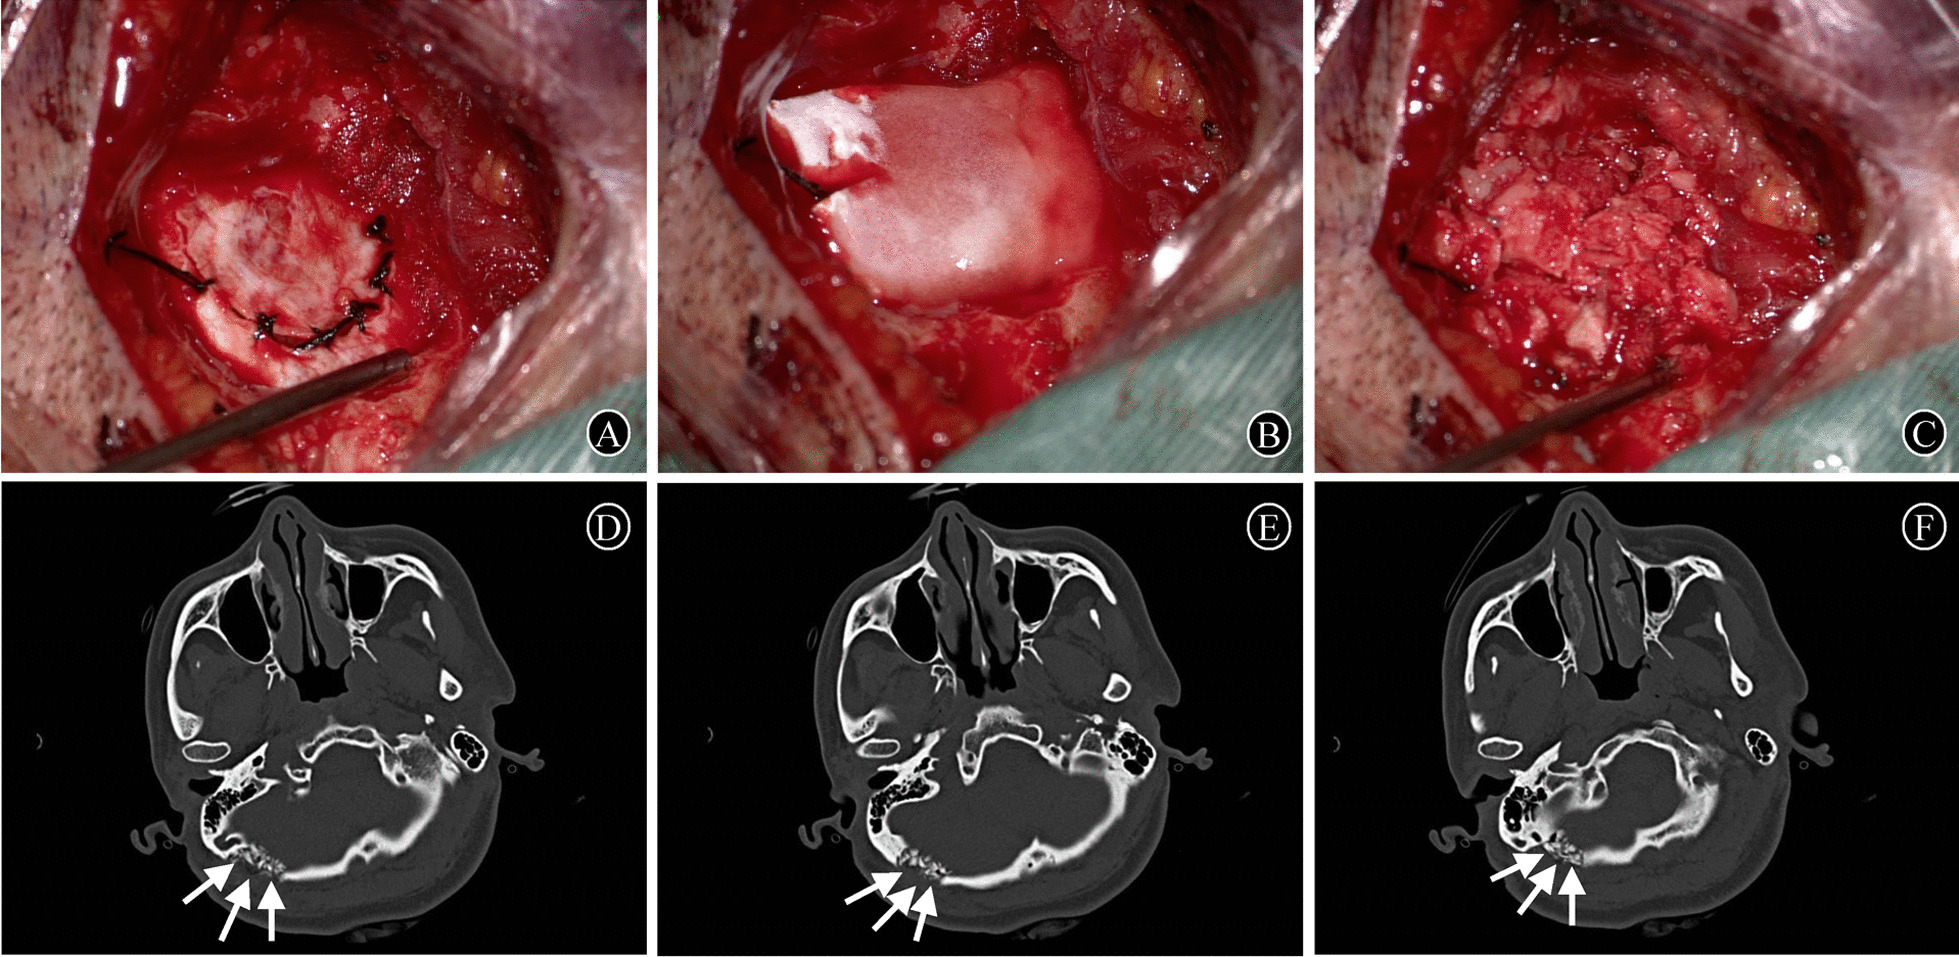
Intraoperative procedure of skull reconstruction and cranial CT results on the first postoperative day. A The dura was closed with watertight sutures after completion of the intracranial microvascular decompression procedure. B A layer of gelatin sponge was placed over the sutured dura. C The autologous skull fragments were placed so that they evenly covered the gelatin sponge. D, E, F The patient’s cranial CT on the first postoperative day showed that the autologous bone fragments filled in the skull defect evenly (white arrow)
